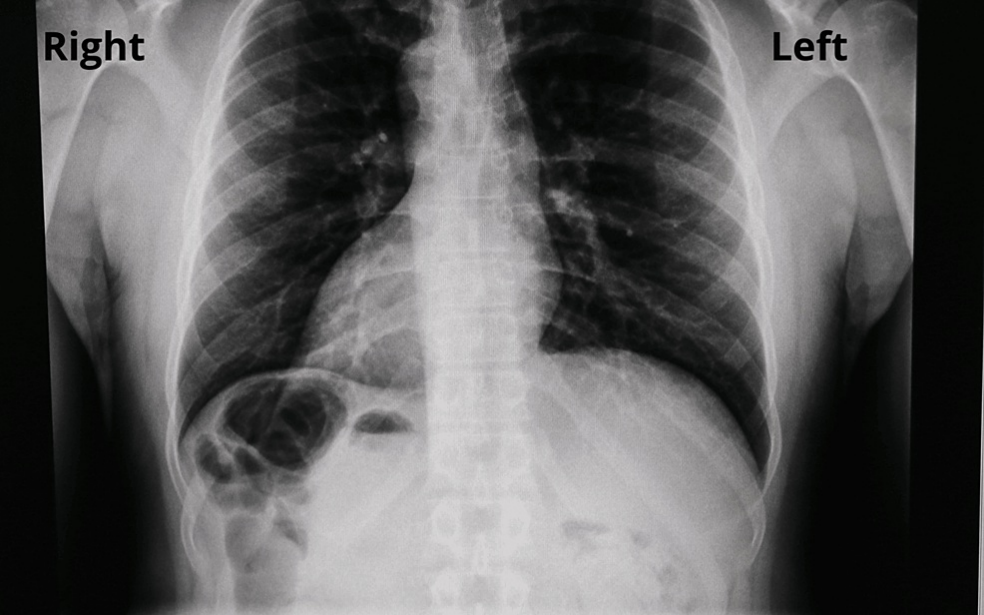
Chest X-ray. Organs are found in an inverted position.
